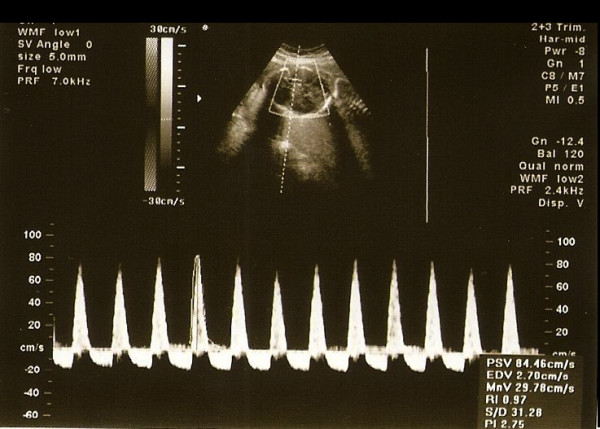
Sonogram showing reverse flow in the MCA
